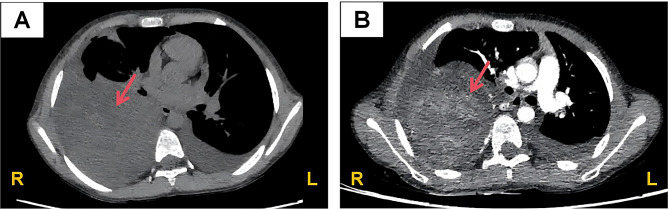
Computerized tomography (CT) images of the child. (A) Representative images of chest CT scan; (B) Representative images of chest enhanced CT scans
